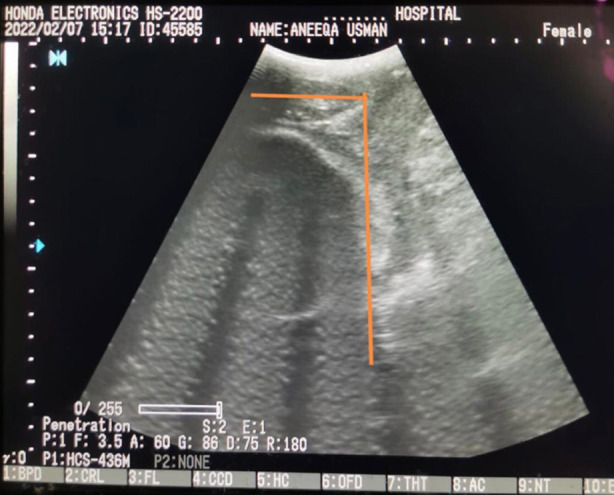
Ultrasound image showing angle of progression.
